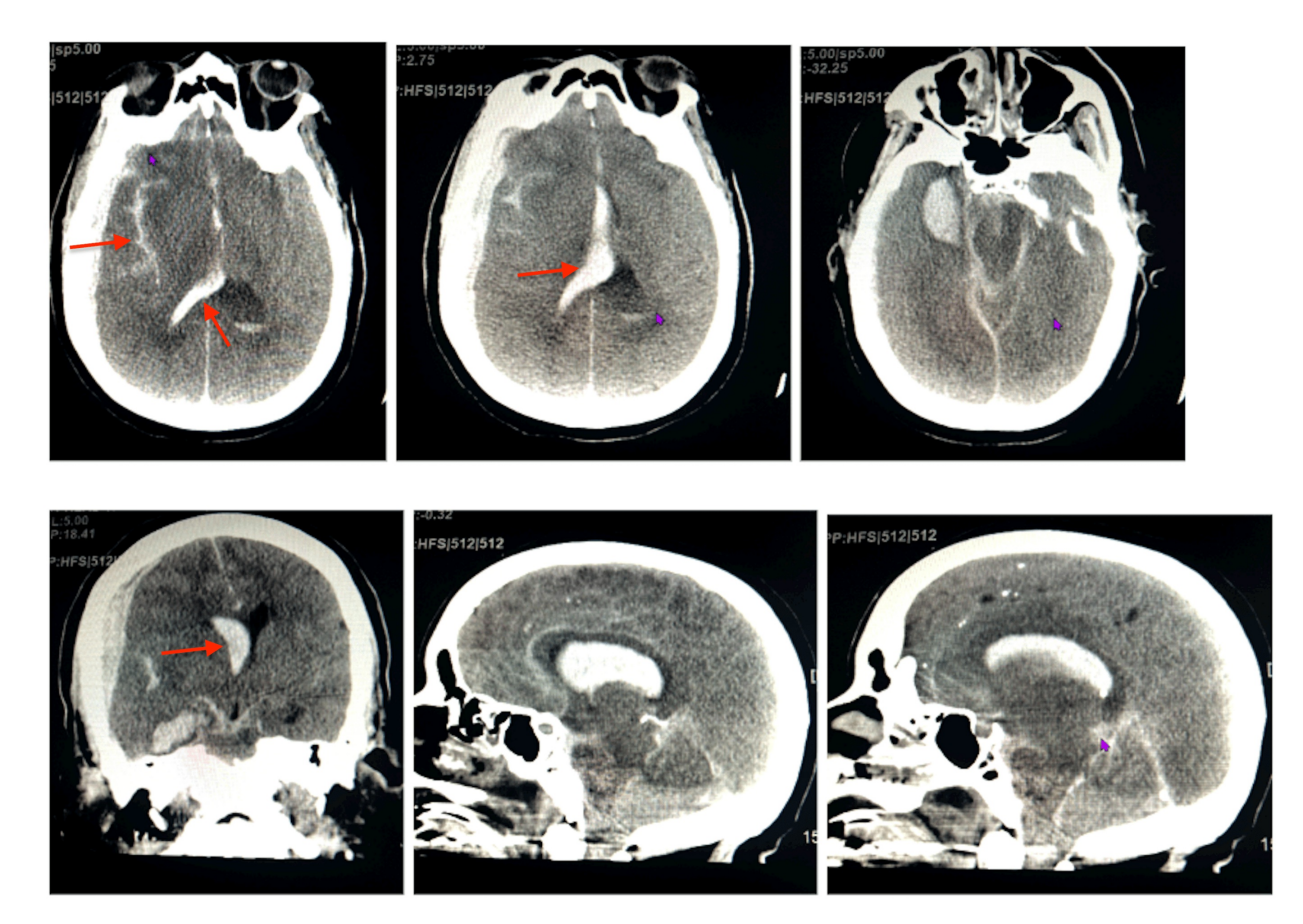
CT head without contrast showing massive hemorrhage
